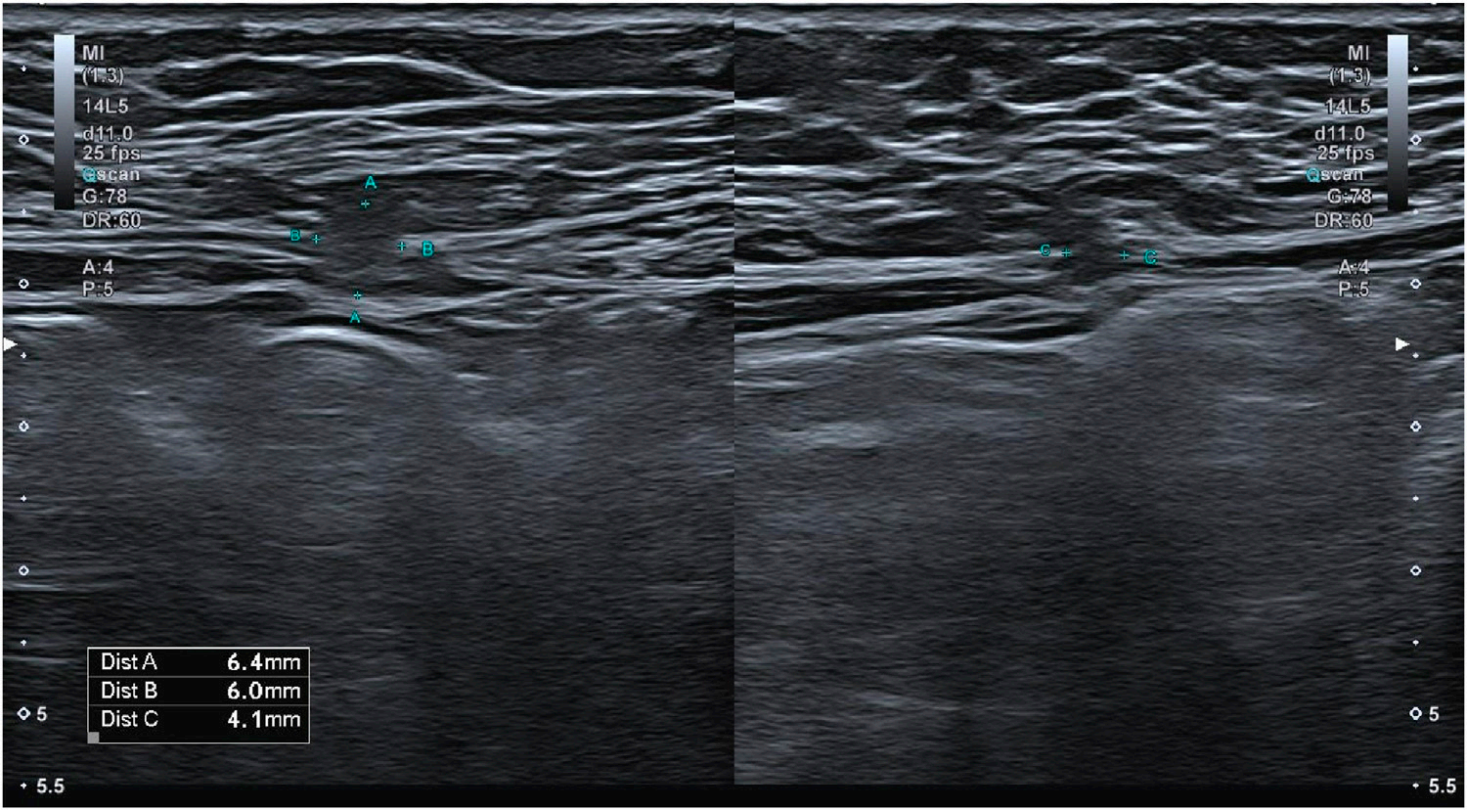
Abdominal wall visualization in ultrasound with TSH.
